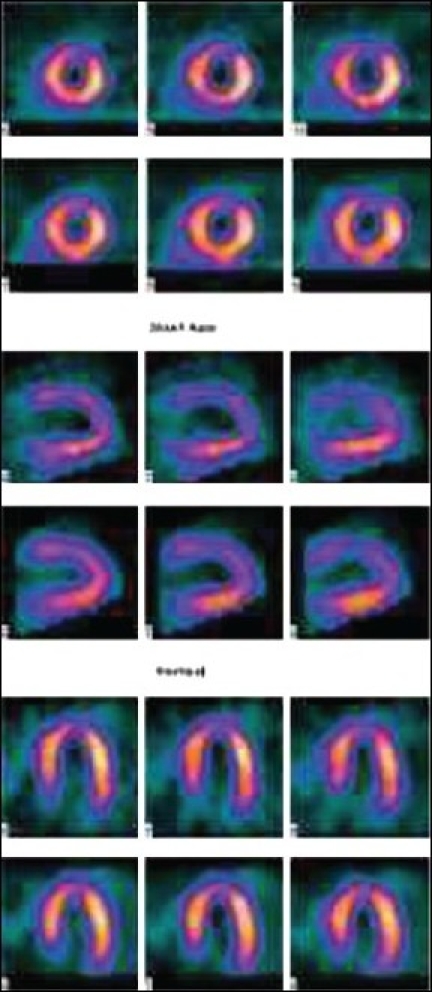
Stress and rest single photon emission computed tomography images of an asymptomatic adolescent boy with uncorrected ALCAPA syndrome showing left anterior descending artery territory ischemia in the background of an old infarct
